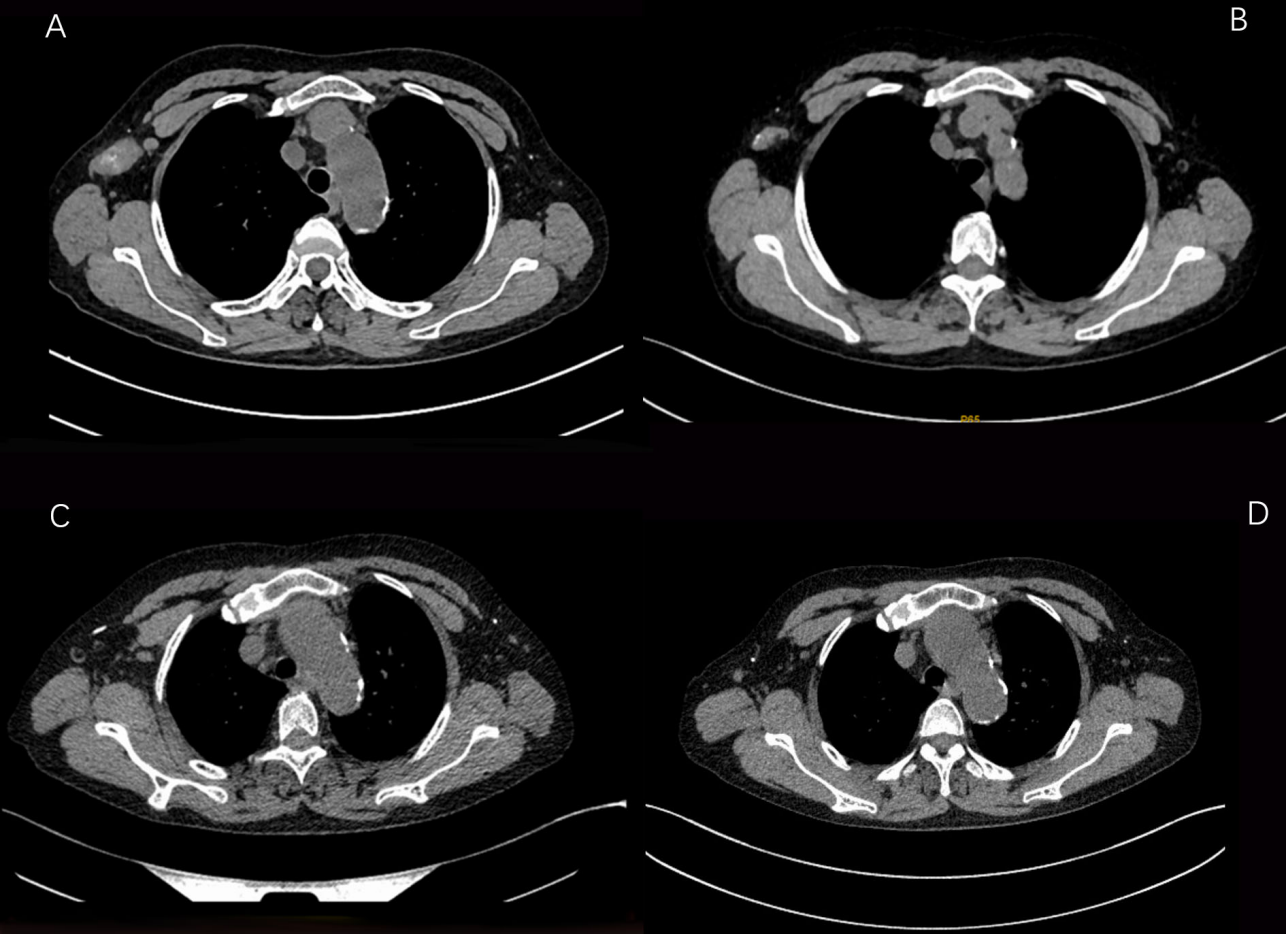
CT images of changes in the axillary lymph node lesions of the patient during the treatment period from August 6, 2021 to February 25, 2022 (A:2021-08-06 B:2021-09-25 C:2021-12-17 D: 2021-02-25).
